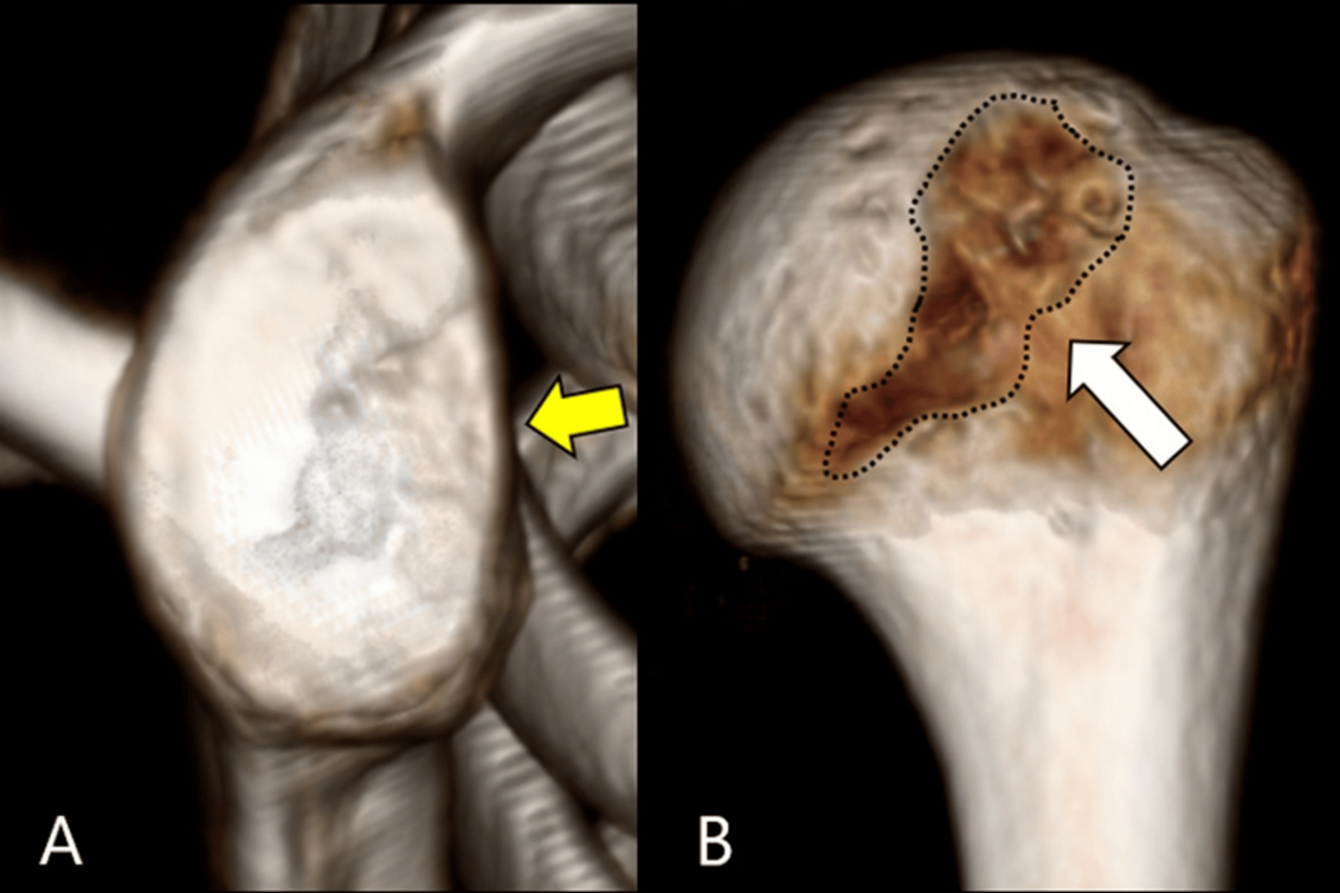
Three-dimensional CT images of the glenoid (A) and humeral head (B) The three-dimensional CT images showed a small glenoid defect (yellow arrow) and a medium-sized Hill-Sachs lesion in the posterosuperior part of the humeral head (white arrow). CT: computed tomography
